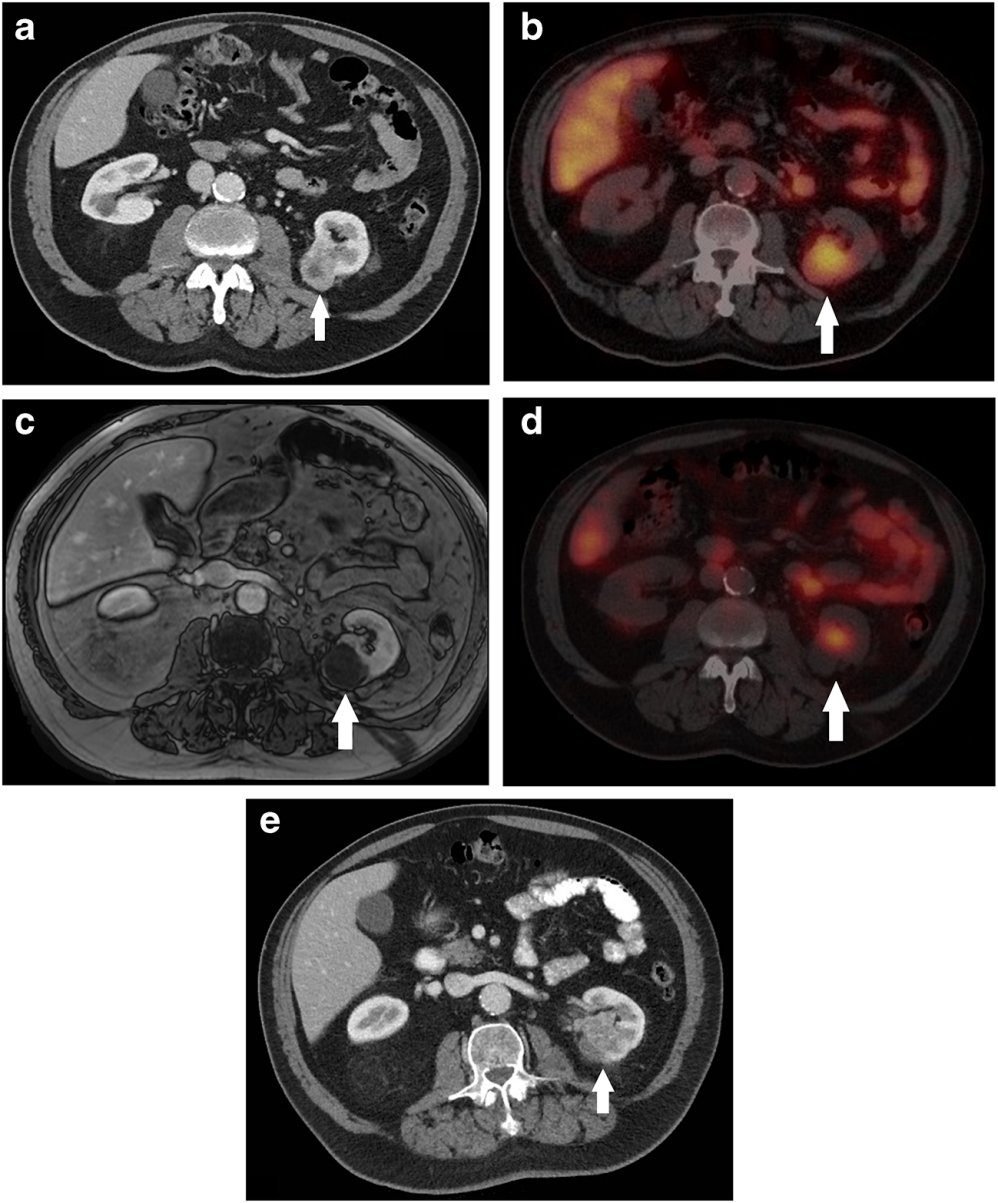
a. In this 79-year-old patient with one known osseous metastasis proven to be clear cell renal cell carcinoma on biopsy, the 32-mm-large primary lesion, as seen on this contrast enhanced axial CT image in the corticomedullary phase (white arrow), was treated with cryoablation. b. The axial preoperative SPECT shows uptake of the tumor (white arrow). c. Axial follow-up MR image at 1 month FU (dynamic contrast-enhanced fat-saturated T1-weighted VIBE sequence) showed no contrast enhancement of the ablated lesion in the corticomedullary phase suggestive for vital tumor presence. d. One month follow-up axial SPECT image showed uptake at the ventral/medial site of the ablated lesion suggestive for residual disease (white arrow). e. Axial 6-month follow-up contrast-enhanced CT image in corticomedullary phase shows nodular contrast enhancement centrally located in the left kidney at the site of the previous ^111^In-girentuximab uptake suggestive for residual/recurrent disease (white arrow)
